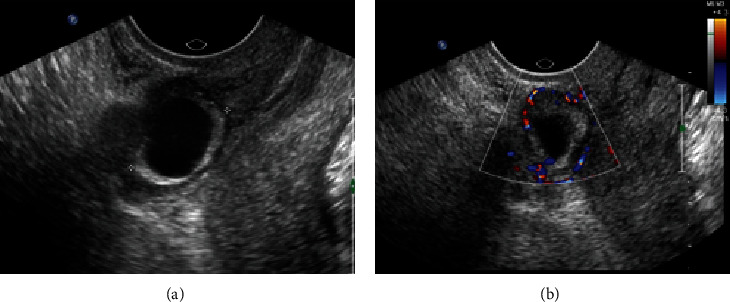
A 29-year-old female (gravida 2, para 1) presented with amenorrhea for 44 days. (a) The sagittal grayscale image showed that the sac was implanted in the lower endometrial cavity, protruding into the scar. A small amount of fluid was seen around the posterior of the conceptus, suggesting that the sac was implanted into the scar. (b) The color Doppler image showed the trophoblastic blood flow surrounding the sac, which made it difficult to estimate the source of the trophoblastic blood. Chorionic villi were visible within the scar with a laparoscopy combined with uterine artery embolization.
